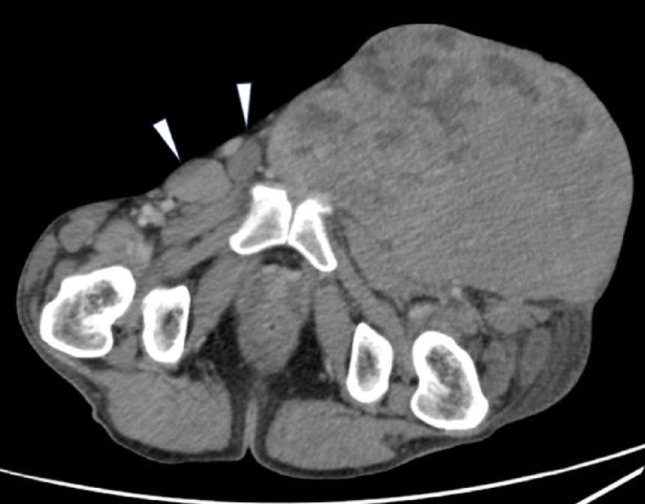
Contrast-enhanced computed tomography shows a heterogenous left testicular tumor measuring 34 × 23 × 16 cm and enlarged bilateral inguinal lymph nodes (arrows, left: 3 × 2 cm, right: 4 × 3 cm)
